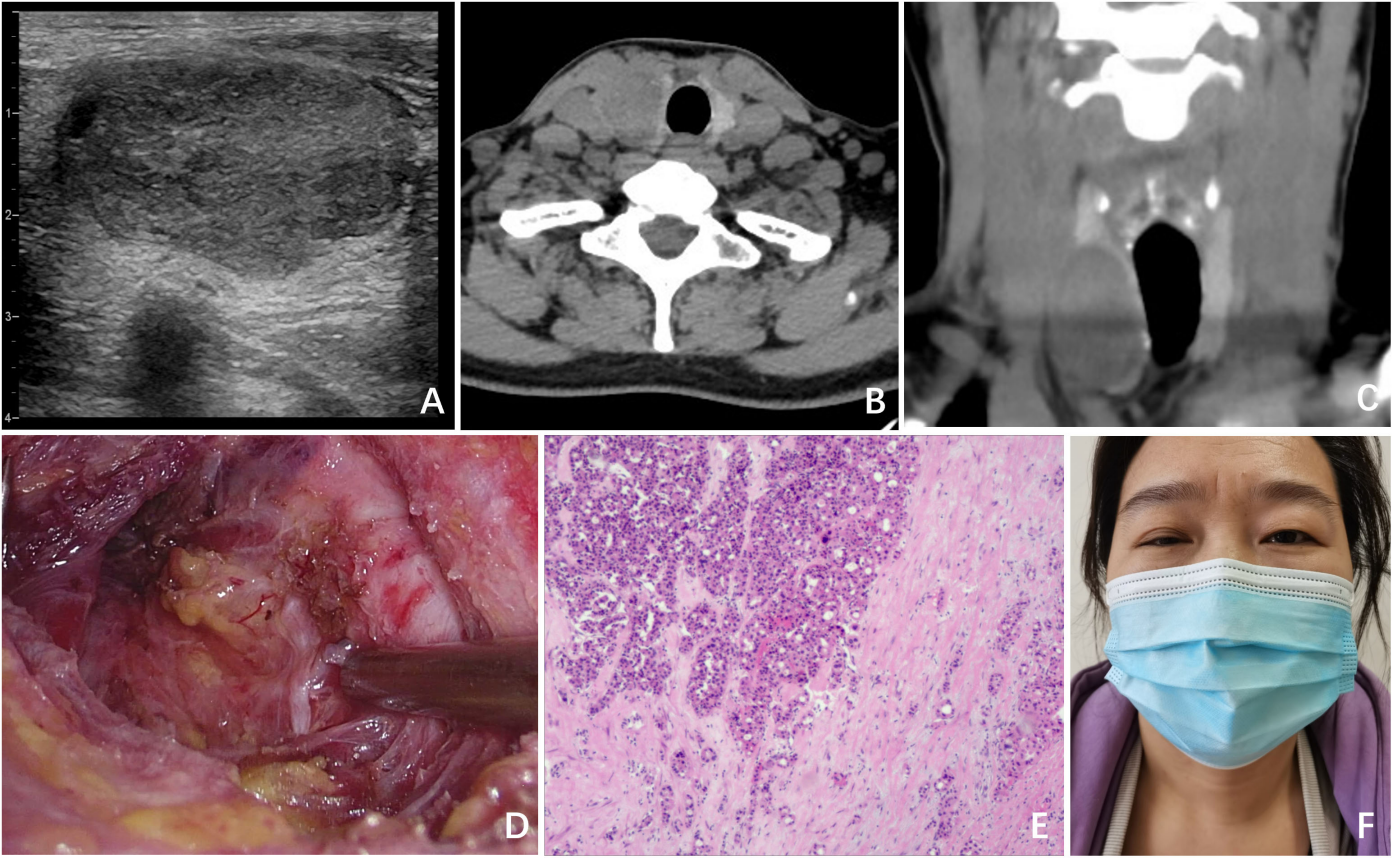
Clinical data of Case 1. (A) Ultrasound revealed a nodule in the right lobe, which had low echogenicity and measured 4.0 cm × 2.4 cm × 2.2 cm; (B) CT (transverse section) revealed a cystic low-density shadow with clear boundaries in the right lobe of the thyroid; (C) CT image of the coronal plane; (D) surgical field after UT by ETS; (E) postoperative pathology indicated thyroid oncocytic adenoma; (F) on POD 1, ptosis of the right eyelid and miosis of the right eye were observed in the patient.
